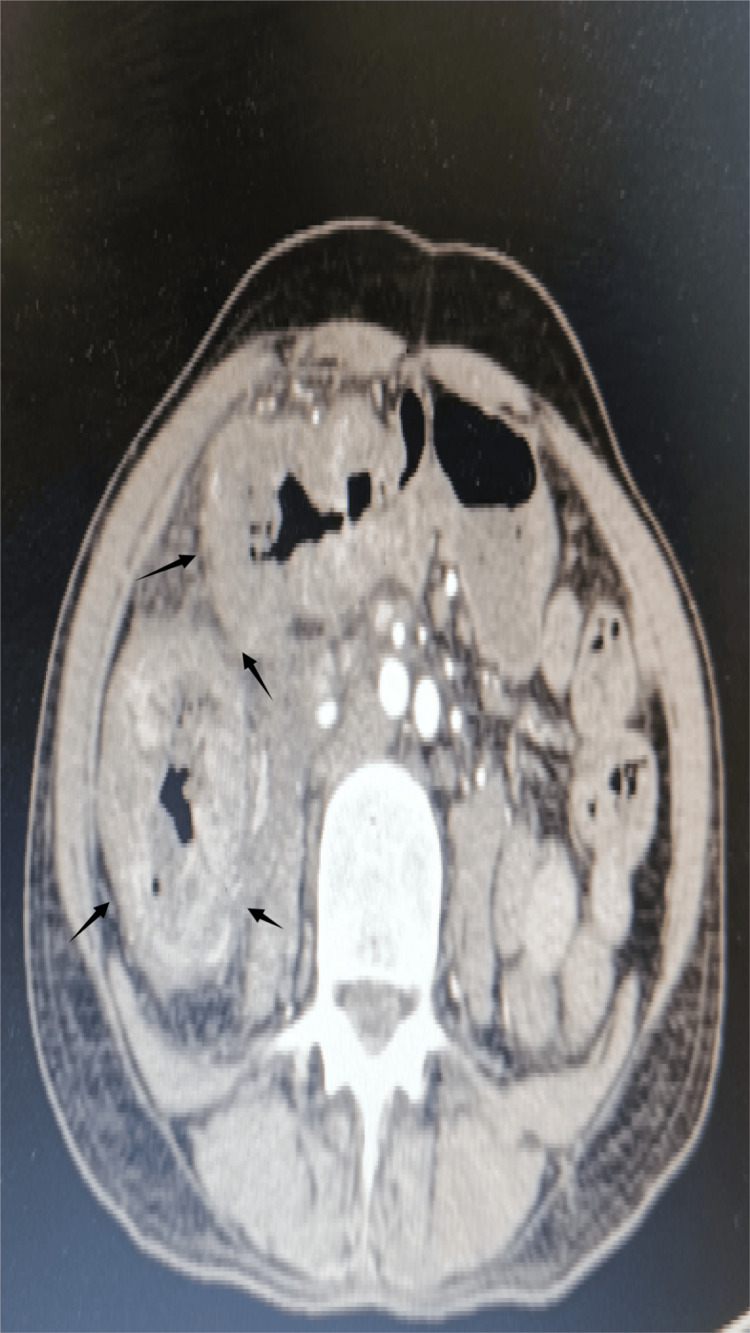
PET-CT demonstrated a locally advanced right colon malignancy with invasion into the pylorus and duodenum PET-CT: positron emission tomography-computed tomography
